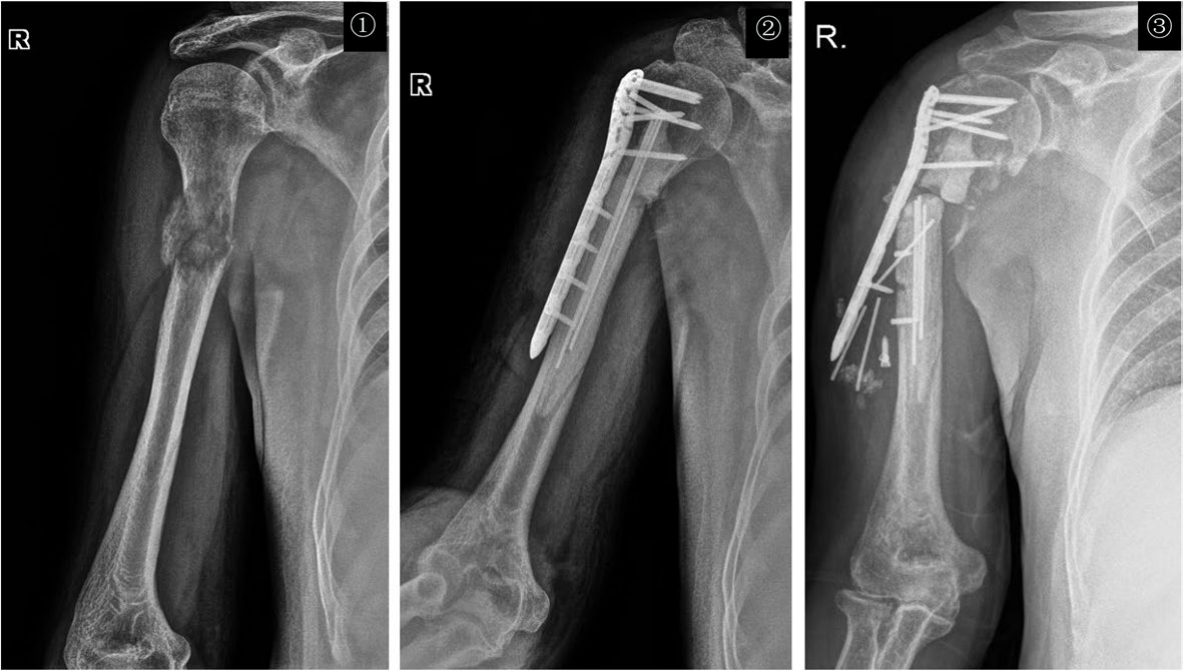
The metastatic tumor of the proximal humerus was treated with curettage and cement fixation, female, 61 years old. ① A positive X-ray of the right humerus showed a pathological fracture of the diaphysis of the humerus due to a metastatic tumor. ② Anteroposterior radiographs of the proximal humerus one month after surgery. ③ Anteroposterior radiographs of the proximal humerus half years after the operation showed a fracture of the proximal humerus and the hardware failure
